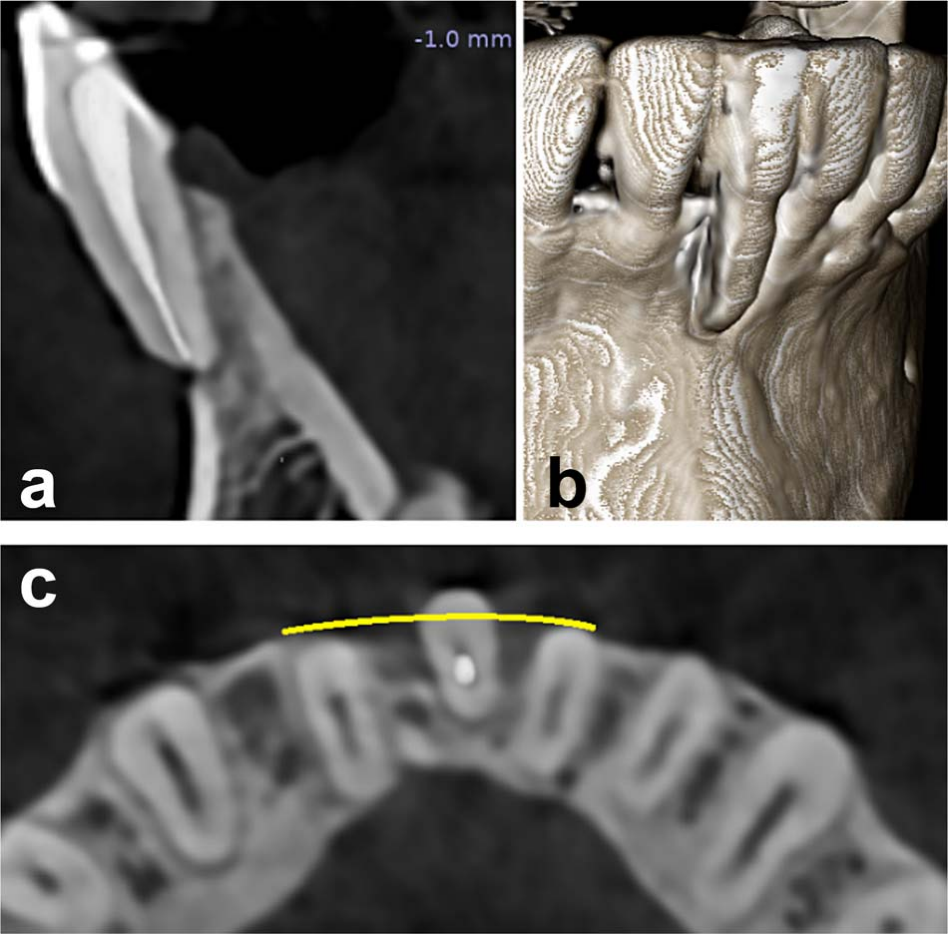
Preoperative radiographical status, (a) sagittal section, (b) 3D construction view, and (c) axial section.
